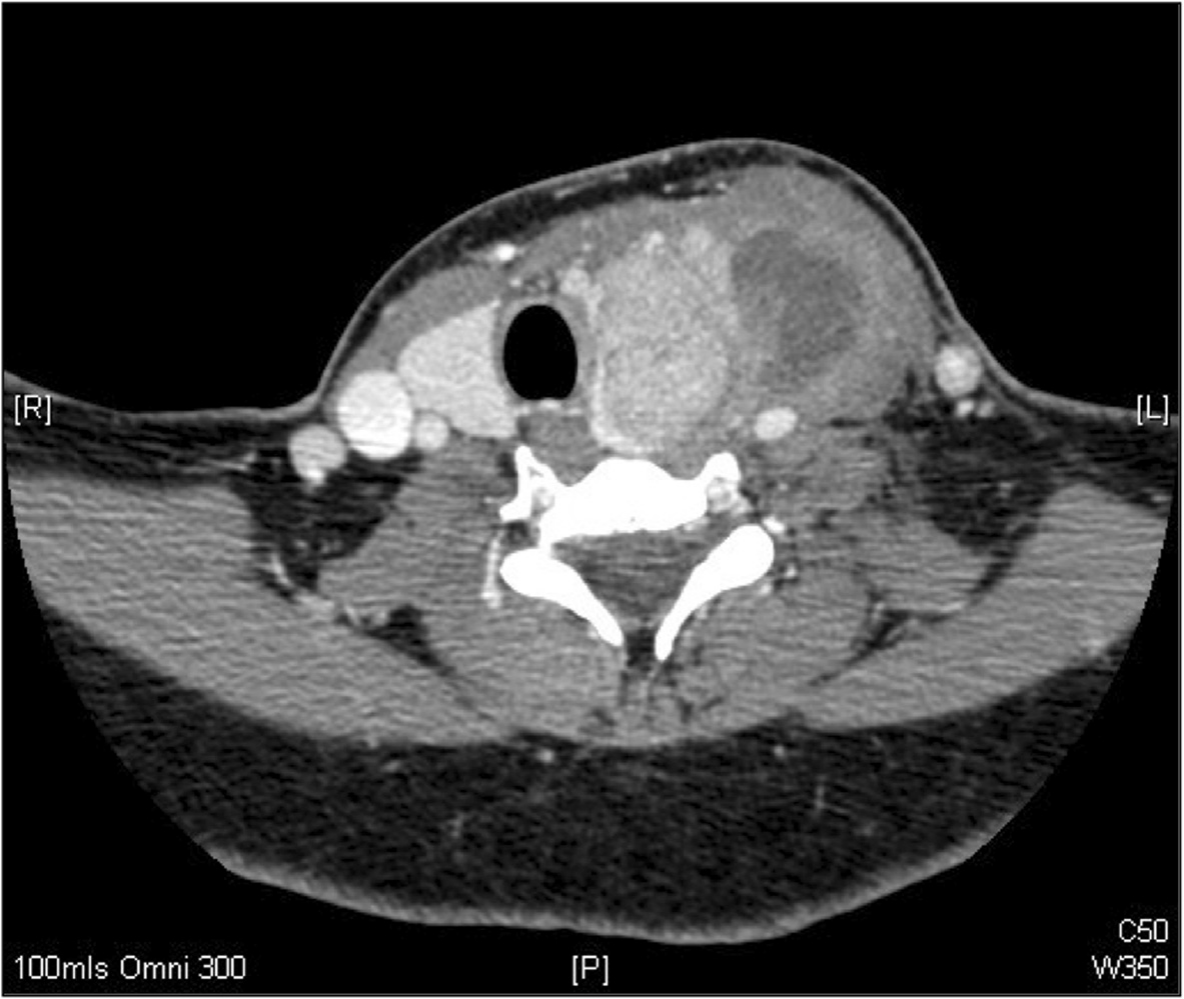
CT with contrast axial slice through neck. CT image demonstrating a large fluid collection arising from left thyroid mass, suggestive of an abscess. Noted compression and deviation of adjacent organs.
